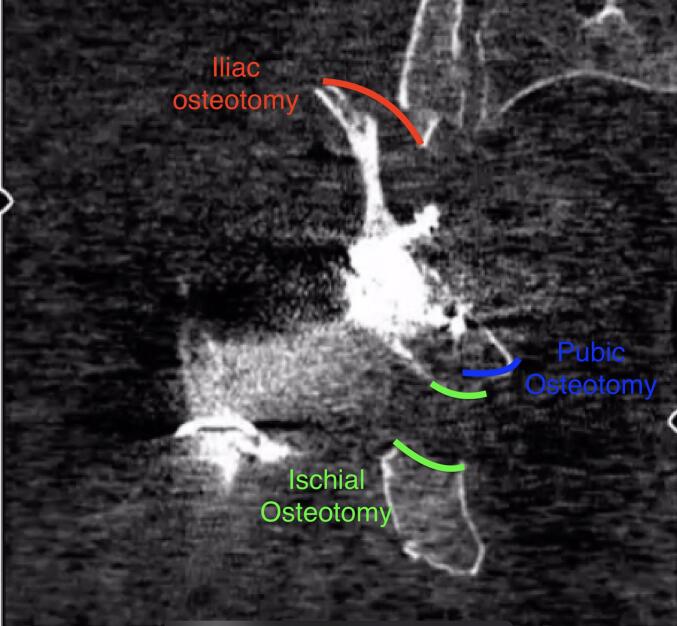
Iliac, pubic and ischial osteotomies marked in the frontal CT section.
